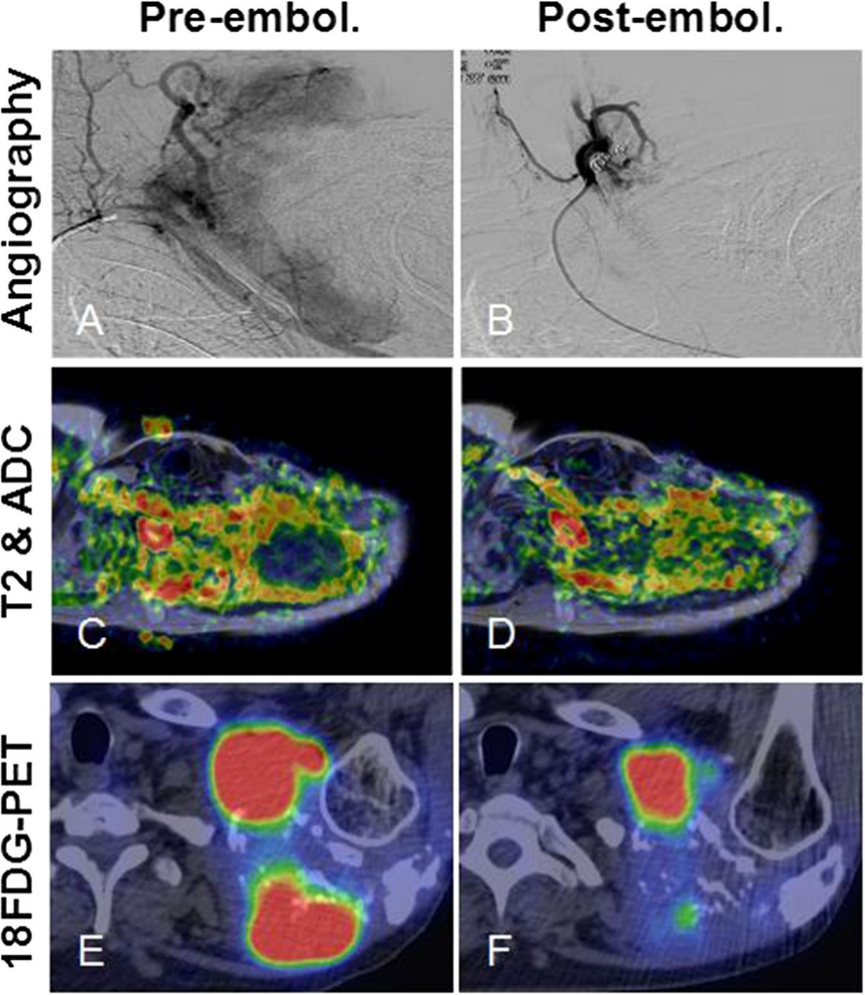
Post-embolization tumor response in the shoulder plasmacytoma could be best demonstrated with functional imaging studies. (A) Angiogram of the left shoulder revealed a well-vascularized lesion, which was supplied by branches from the left subclavian artery. (B) Selective catheterization of the feeding arteries and embolization of the tumor vasculature with 350 to 500 μm PVA particles were performed in two sessions from a right femoral puncture and a left brachial puncture, respectively. Some arterial branches were also closed off with coils. DWIBS MRI proved to be a sensitive modality for locating densely packed foci of tumor cells inside a heterogeneous lesion. (C) We co-registered ADC maps with T2 weighted images that allowed more precise comparison between baseline and follow-up scans. (D) The increase of ADC values by 4 weeks post-embolization was clearly visible on the fusion map. (E) Some DWIBS restricted foci also showed high ^18^FDG uptake on PET-CT. (F) Following embolization, decreased metabolic activity was detected, which was inversely correlated with increased diffusivity, a sign of tumor necrosis.
